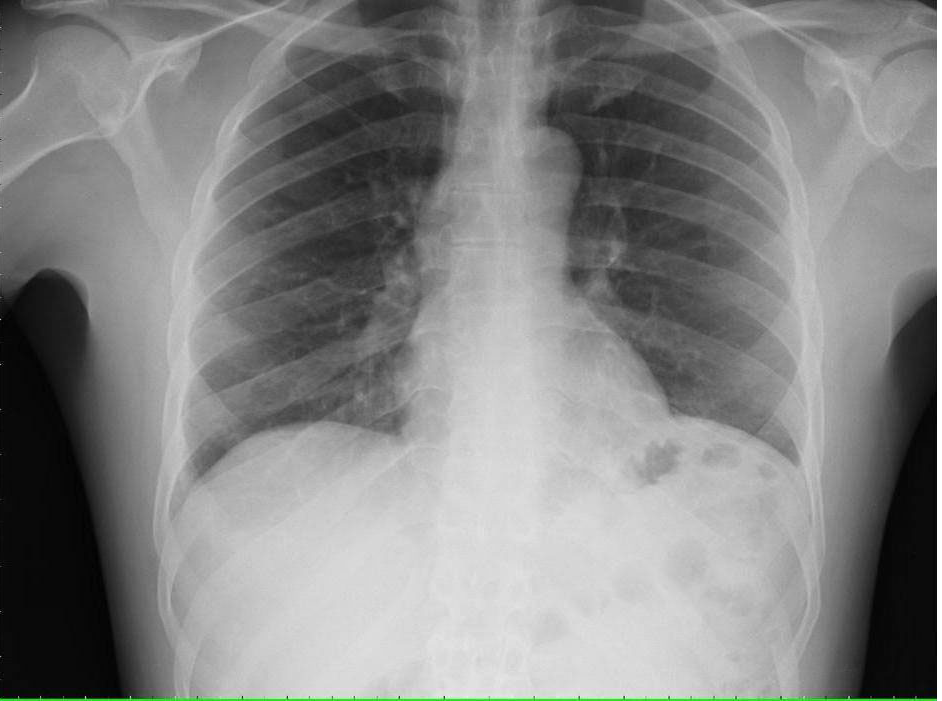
Chest x-ray showing no evidence of active pulmonary disease.
